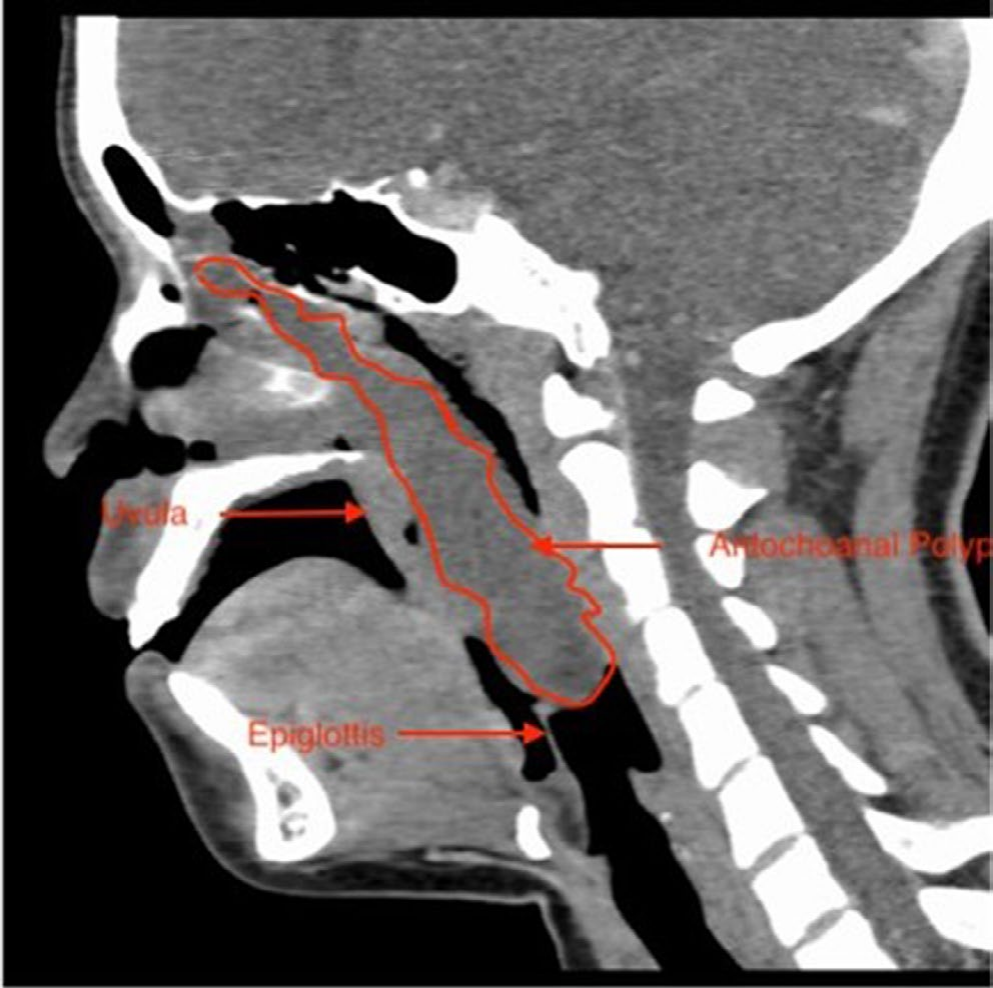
Sagittal CT image showing the extent of airway obstruction caused by the polyp
